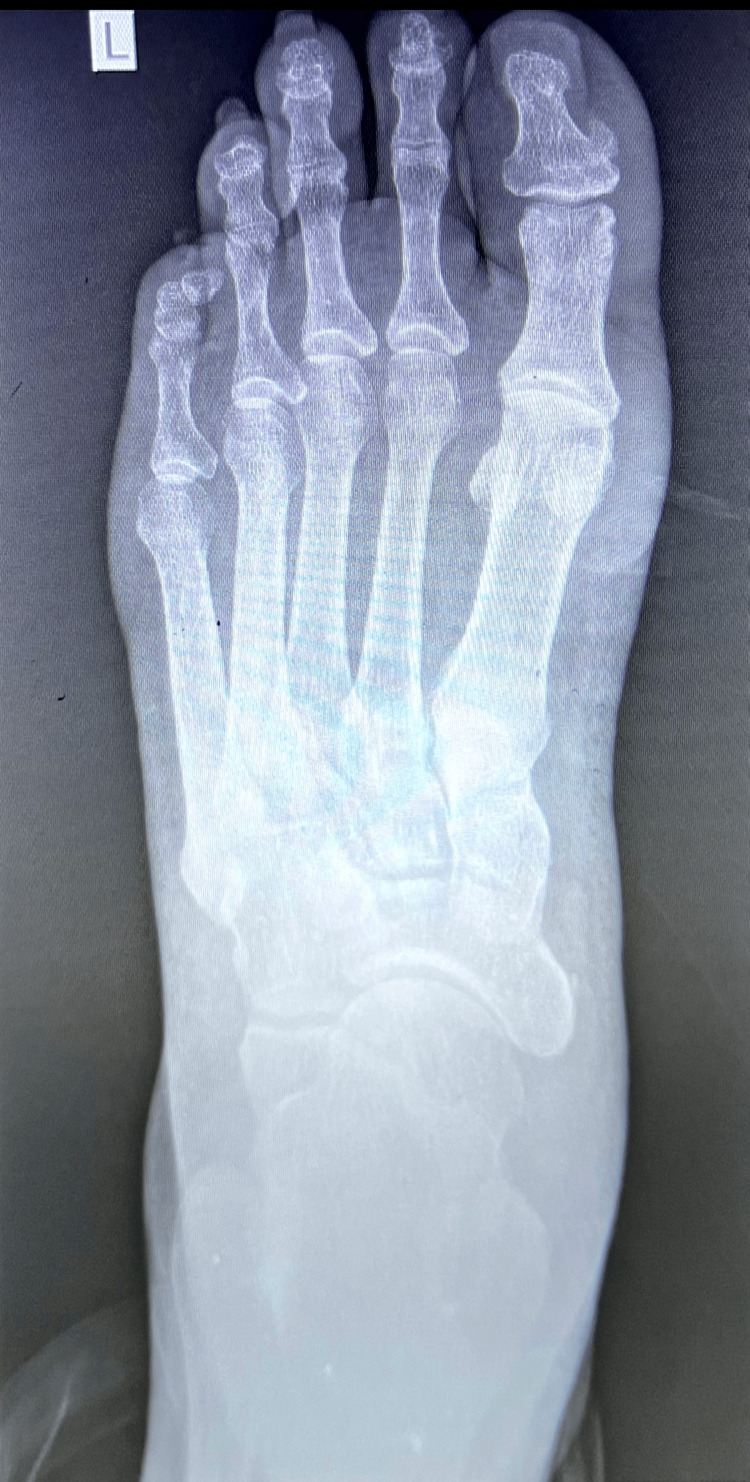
Follow‑up AP plain X-ray of the left foot at four weeks post‑injury showing no fractures. AP: anteroposterior.
